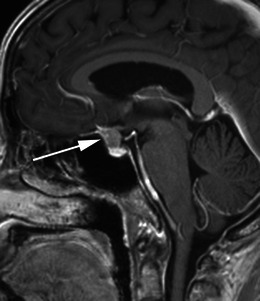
Post-contrast sagittal T1-weighted sequence in a 52-year-old woman with reduced vision in the left eye demonstrates a homogeneously enhancing suprasellar mass (*arrow*) extending inferiorly into the superior aspect of the sella where it contacts the brightly enhancing anterior pituitary. The mass results in mild elevation of the left side of the optic chiasm
